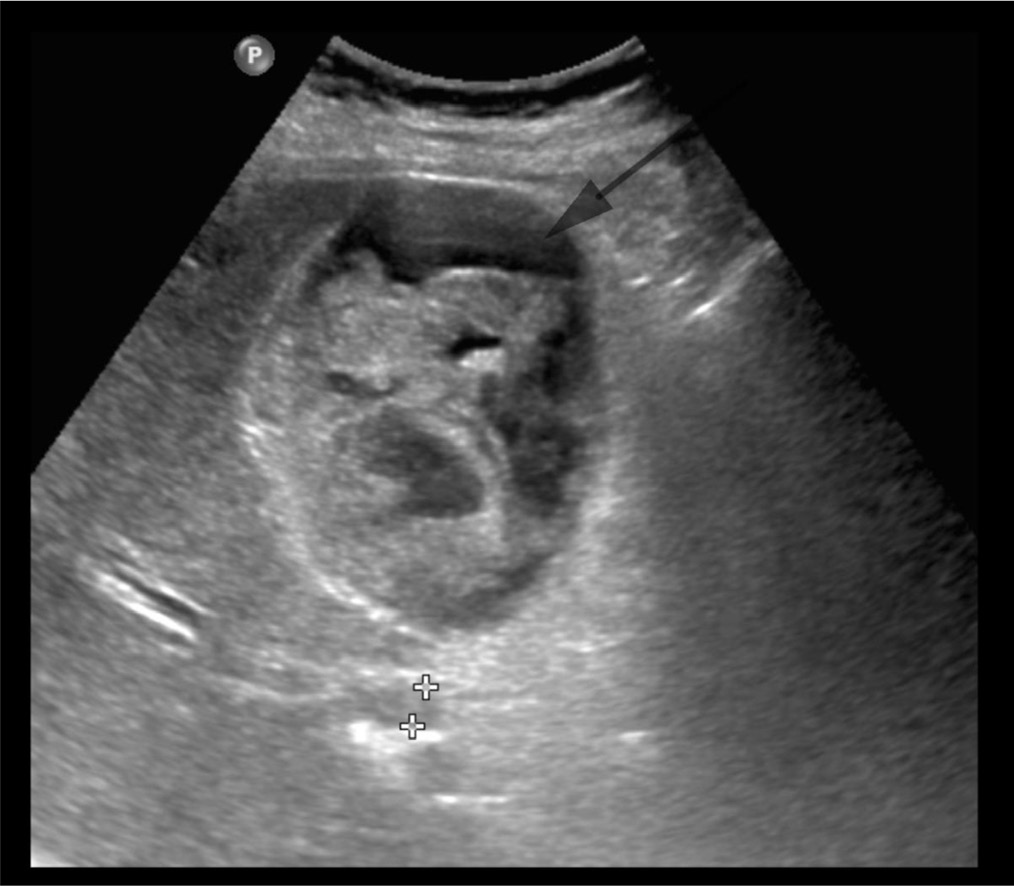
Ultrasound abdomen and pelvis
